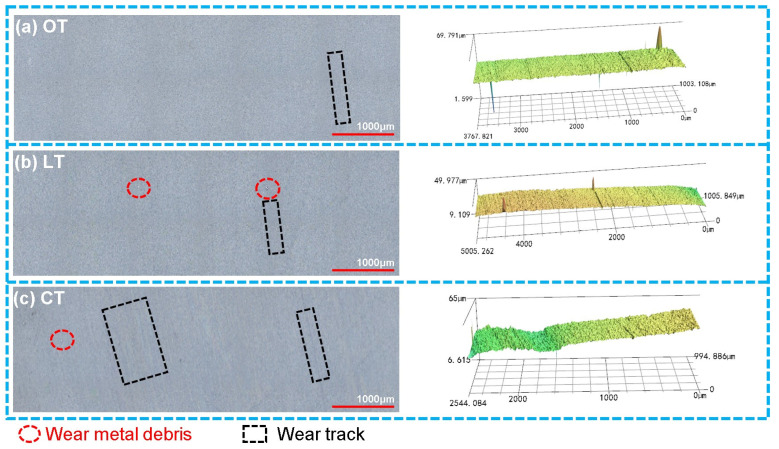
Representative worn surfaces and 3D morphologies of the PTFE rings of OT, LT and CT after ultrasonic cleaning. (a) OT; (b) LT; (c) CT.
